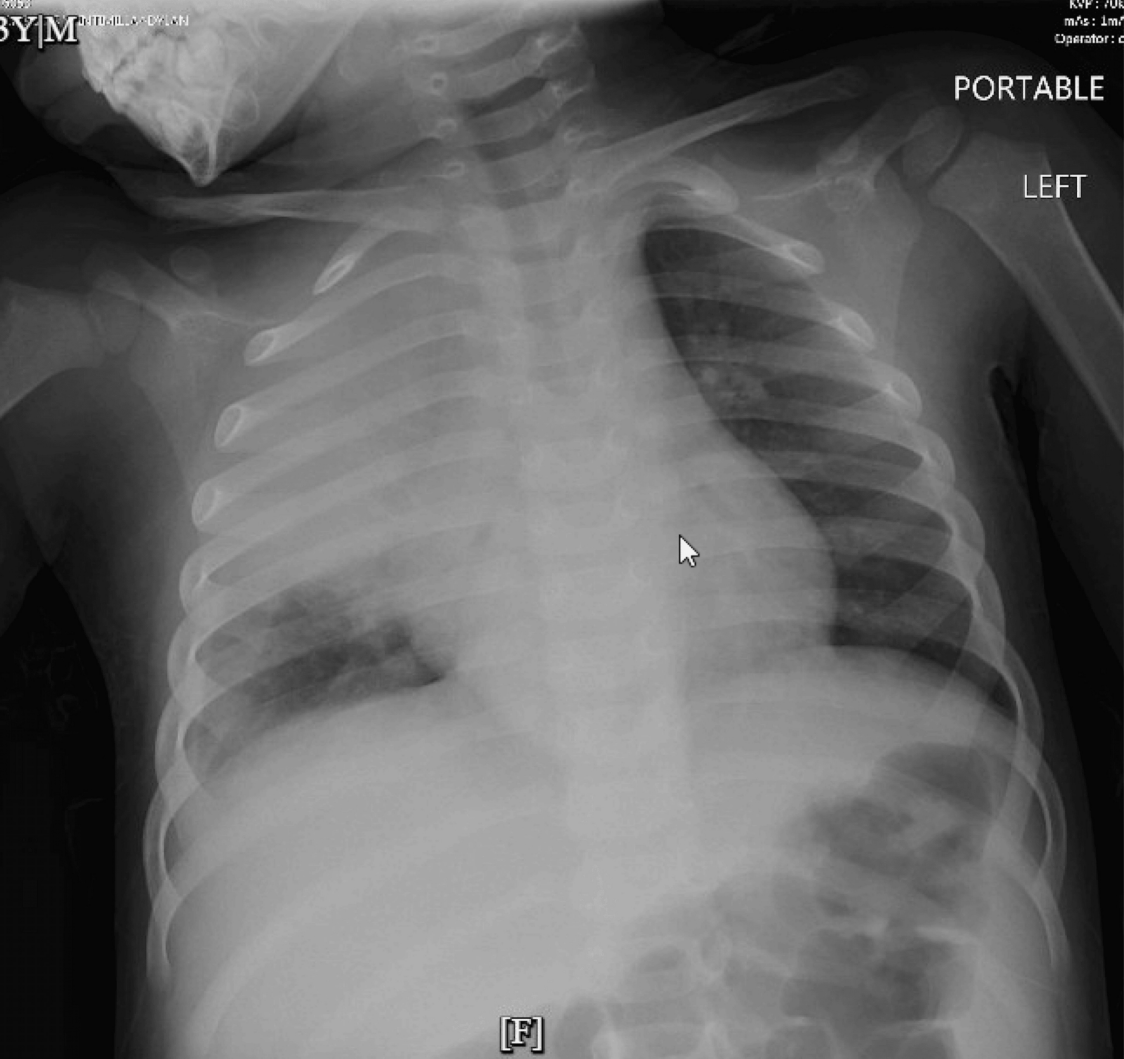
Chest X-ray, hospital day 1; dense opacity of the right mid to upper hemithorax may represent loculated pleural effusion, pneumonia, and/or atelectasis.
